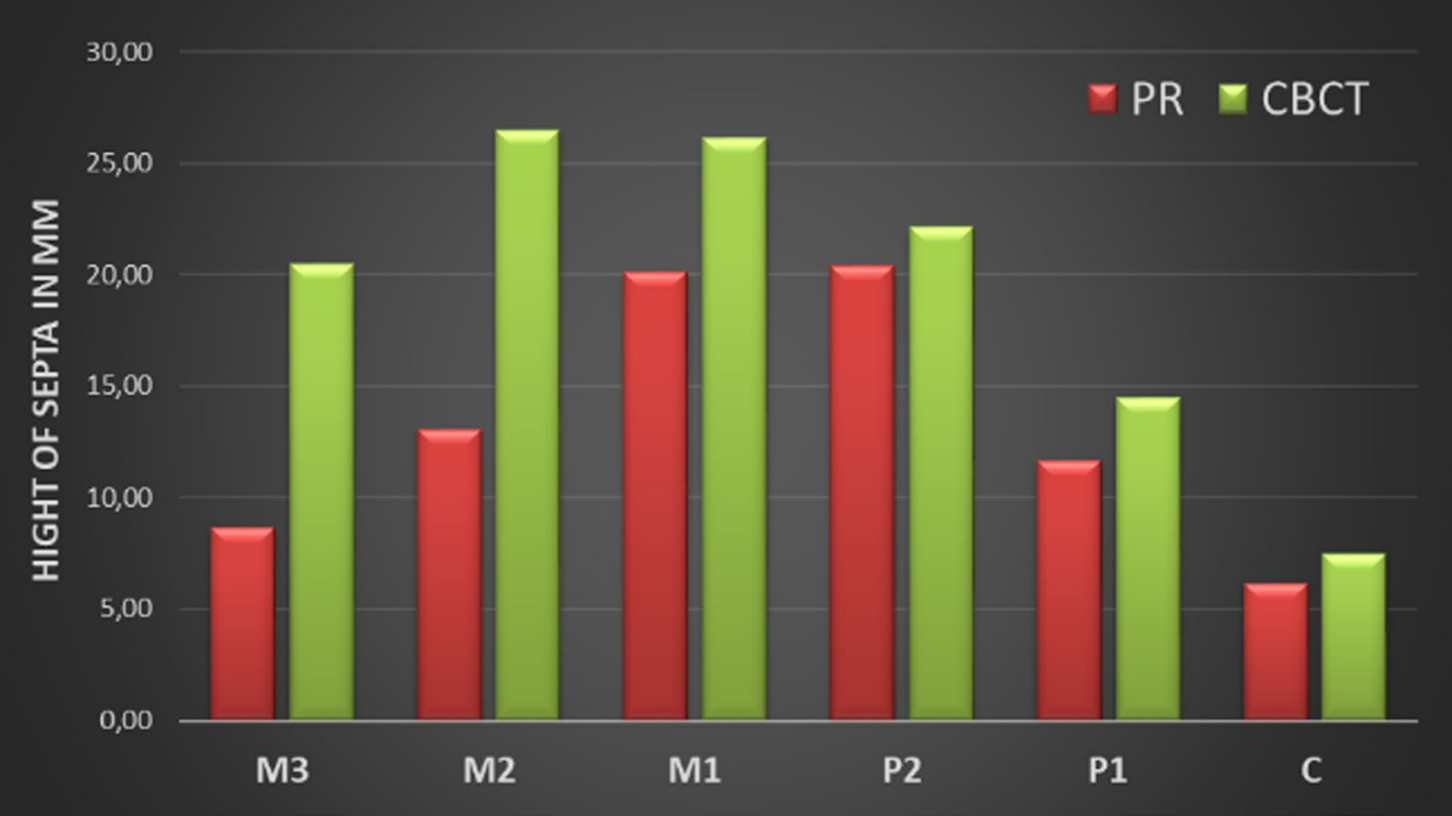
The height of the septa on PR versus CBCT images
